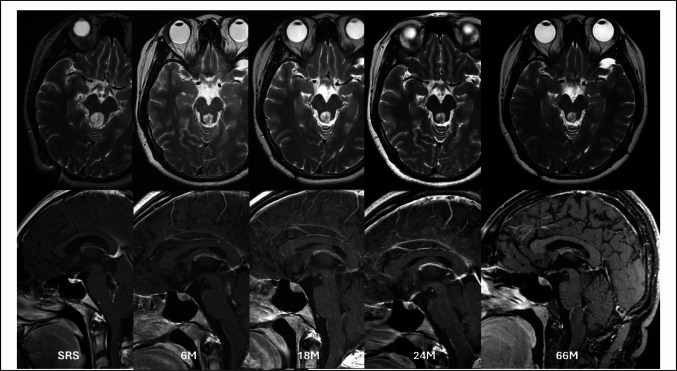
A 24-year-old female patient with a rosette-forming glioneuronal tumor of the fourth ventricle with periaqueductal extension. The patient initially underwent craniotomy for tumor evacuation. Residual tumor was evident on postoperative MRI, measuring 3.86 cm^3^, and she subsequently underwent stereotactic radiosurgery. Over the subsequent two years, the tumor volume continued to regress. The lesion remained stable at the most recent clinical follow-up, 66 months after stereotactic radiosurgery. No radiation-related adverse effects were observed throughout the follow-up period
